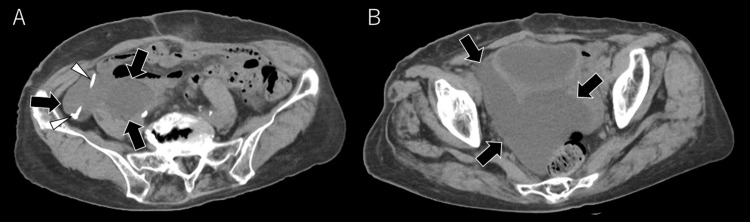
Abdominal CT following shuntography (A, B) Fluid collection is observed from the right paracolic gutter to the vesicorectal pouch (arrows), with the catheter tip within the cyst (arrowheads).
